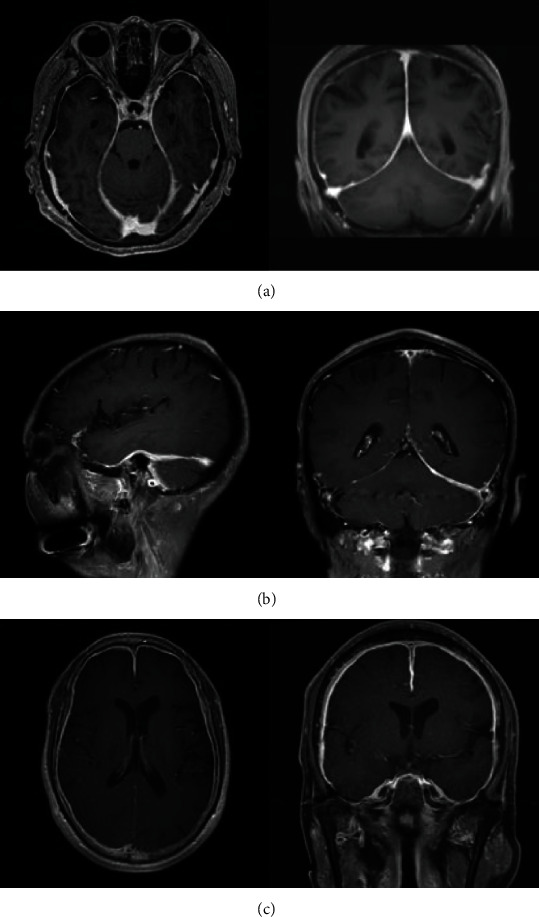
Neuroimaging of hypertrophic pachymeningitis. Case 4. Axial and coronal T1-contrasted MRI reveal enhancement of thickened falx cerebrum and tentorium cerebellum, mimicking the logo of Mercedes Benz (“Benz” sign) in coronal MRI (a). Case 5. Sagittal and coronal T1-contrasted MRI show thickening of left tentorium cerebellum, left frontal, and temporal dura mater (b). Case 6. Axial and coronal T1-contrasted MRI show diffuse linear dural enhancement, including the falx cerebrum (c).
